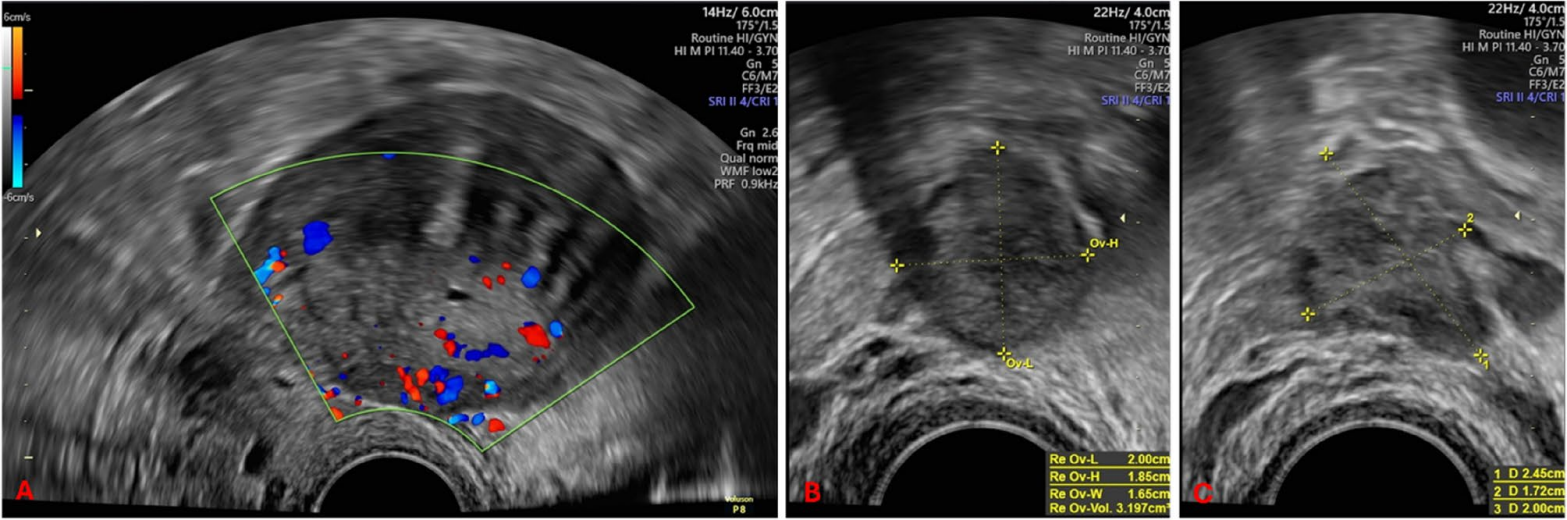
Transvaginal ultrasound imaging of the internal genitalia. A: longitudinal section of the uterus, with suspected uterine adenomyosis; B: normal right ovary; C: normal left ovary
